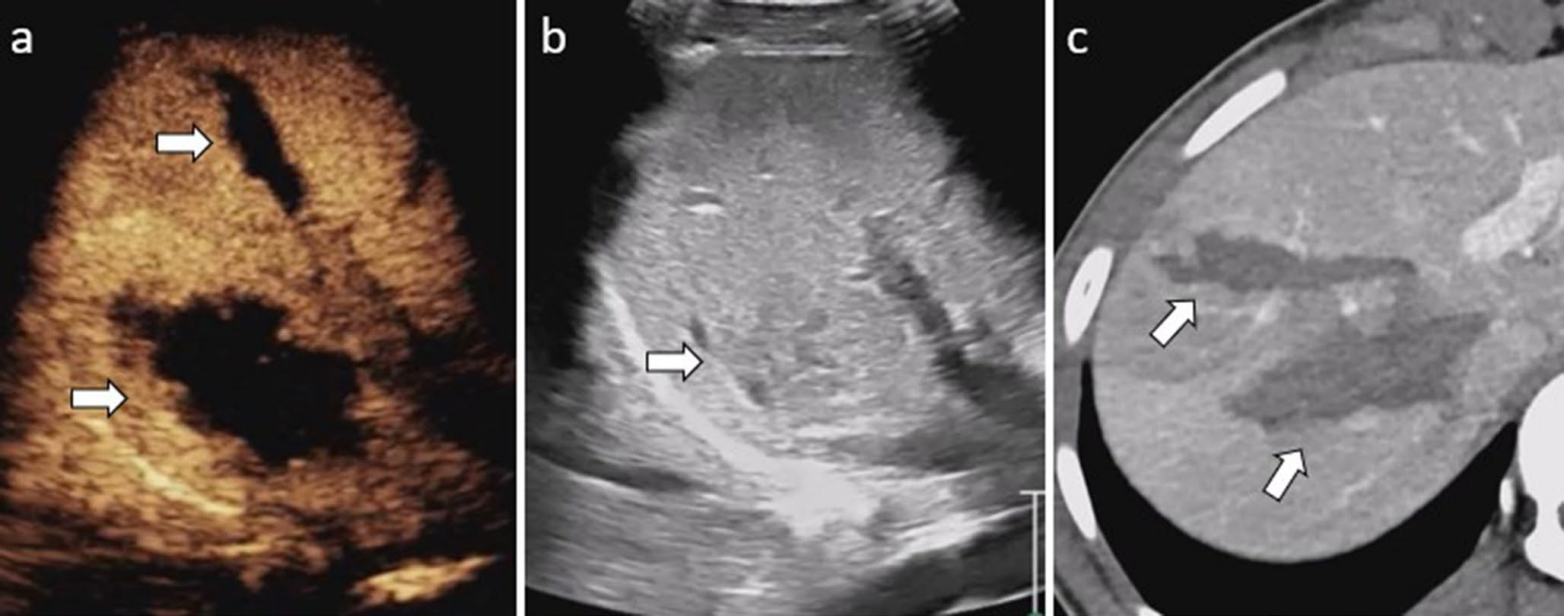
Liver laceration in a pediatric patient. (a) Transverse contrast-enhanced ultrasound (CEUS) of the liver reveals irregular hypoenhancing lacerations (arrows) of the liver in segments 5 and 6. (b) Transverse gray-scale US of the right hepatic lobe demonstrates a subtle decrease in parenchymal echogenicity in the corresponding region (arrow). (c) Axial intravenous-contrast-enhanced CT image confirms the hepatic laceration (arrows)
